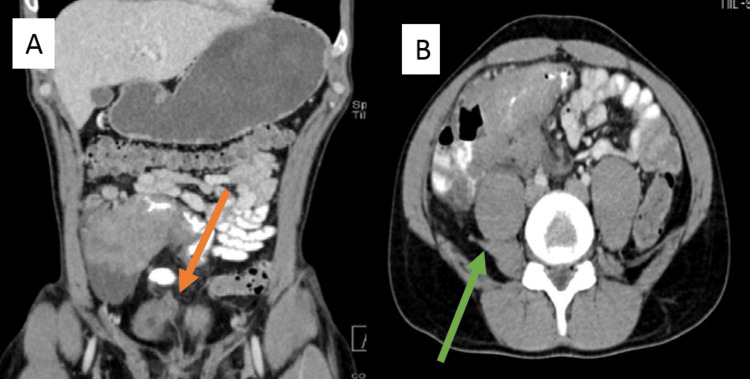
Contrast-enhanced CT scan (Case 1) Contrast-enhanced CT scan (A: coronal plane, B: axial plane) demonstrates marked irregular thickening of the terminal ileum with a mass-like appearance (green arrow) and ileocecal valve (orange arrow), associated with minimal ileocolic mesenteric inflammatory fat stranding. Terminal ileum luminal narrowing secondary to the mural thickening.
